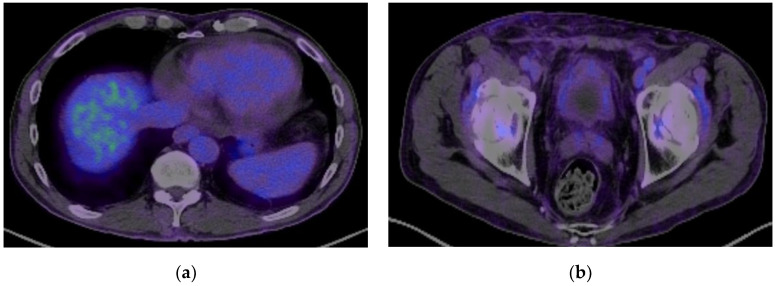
A PET-CT scan showing remission of the pulmonary metastasis (a) and no recurrence of the malignant process (b).
